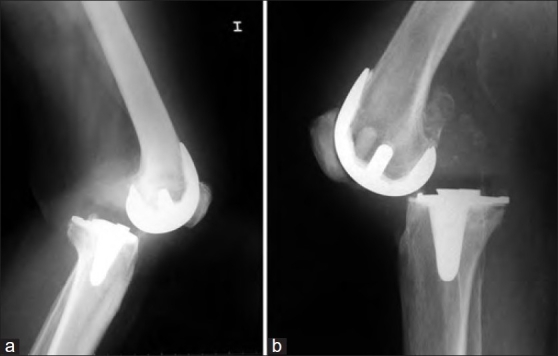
X-ray (lateral view) of knee joint showing posterior dislocation. Patellar tendon has not been avulsed. Imbalanced gap with PCL incompetence were the main contributing factors. Cases 1 and 5
